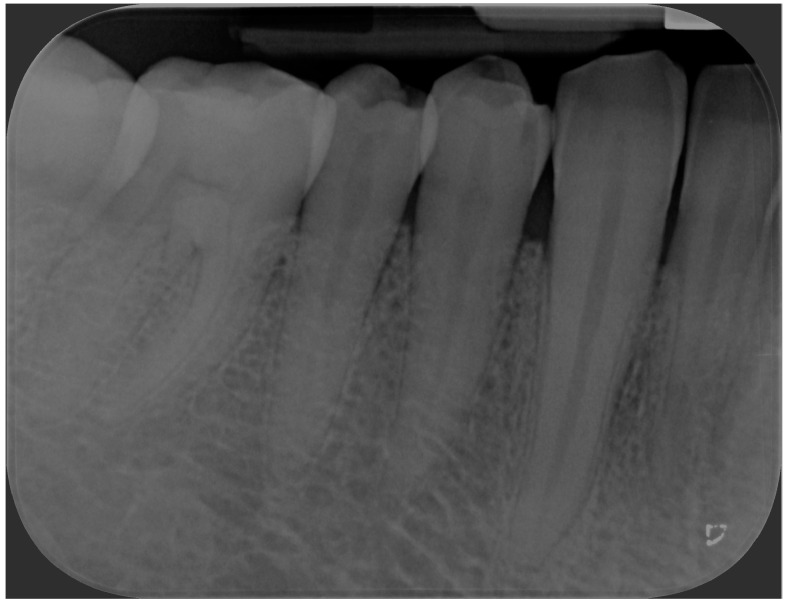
Periapical radiograph of the mandibular premolar right region after 6-months follow-up, showing no pathological alteration of the bone.
